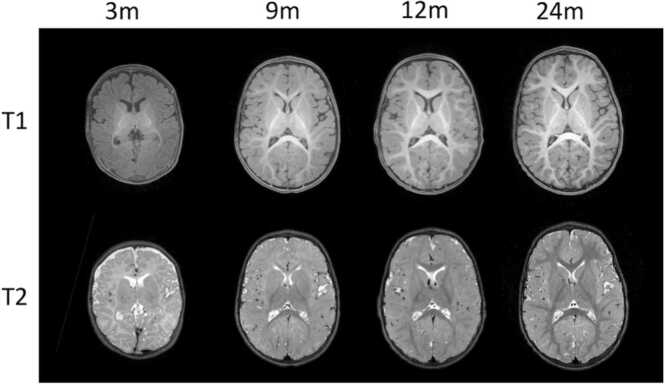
T1- and T2-weighted image contrast from the same individual across the first two postnatal years (scanned at 3, 9, and 12months).
